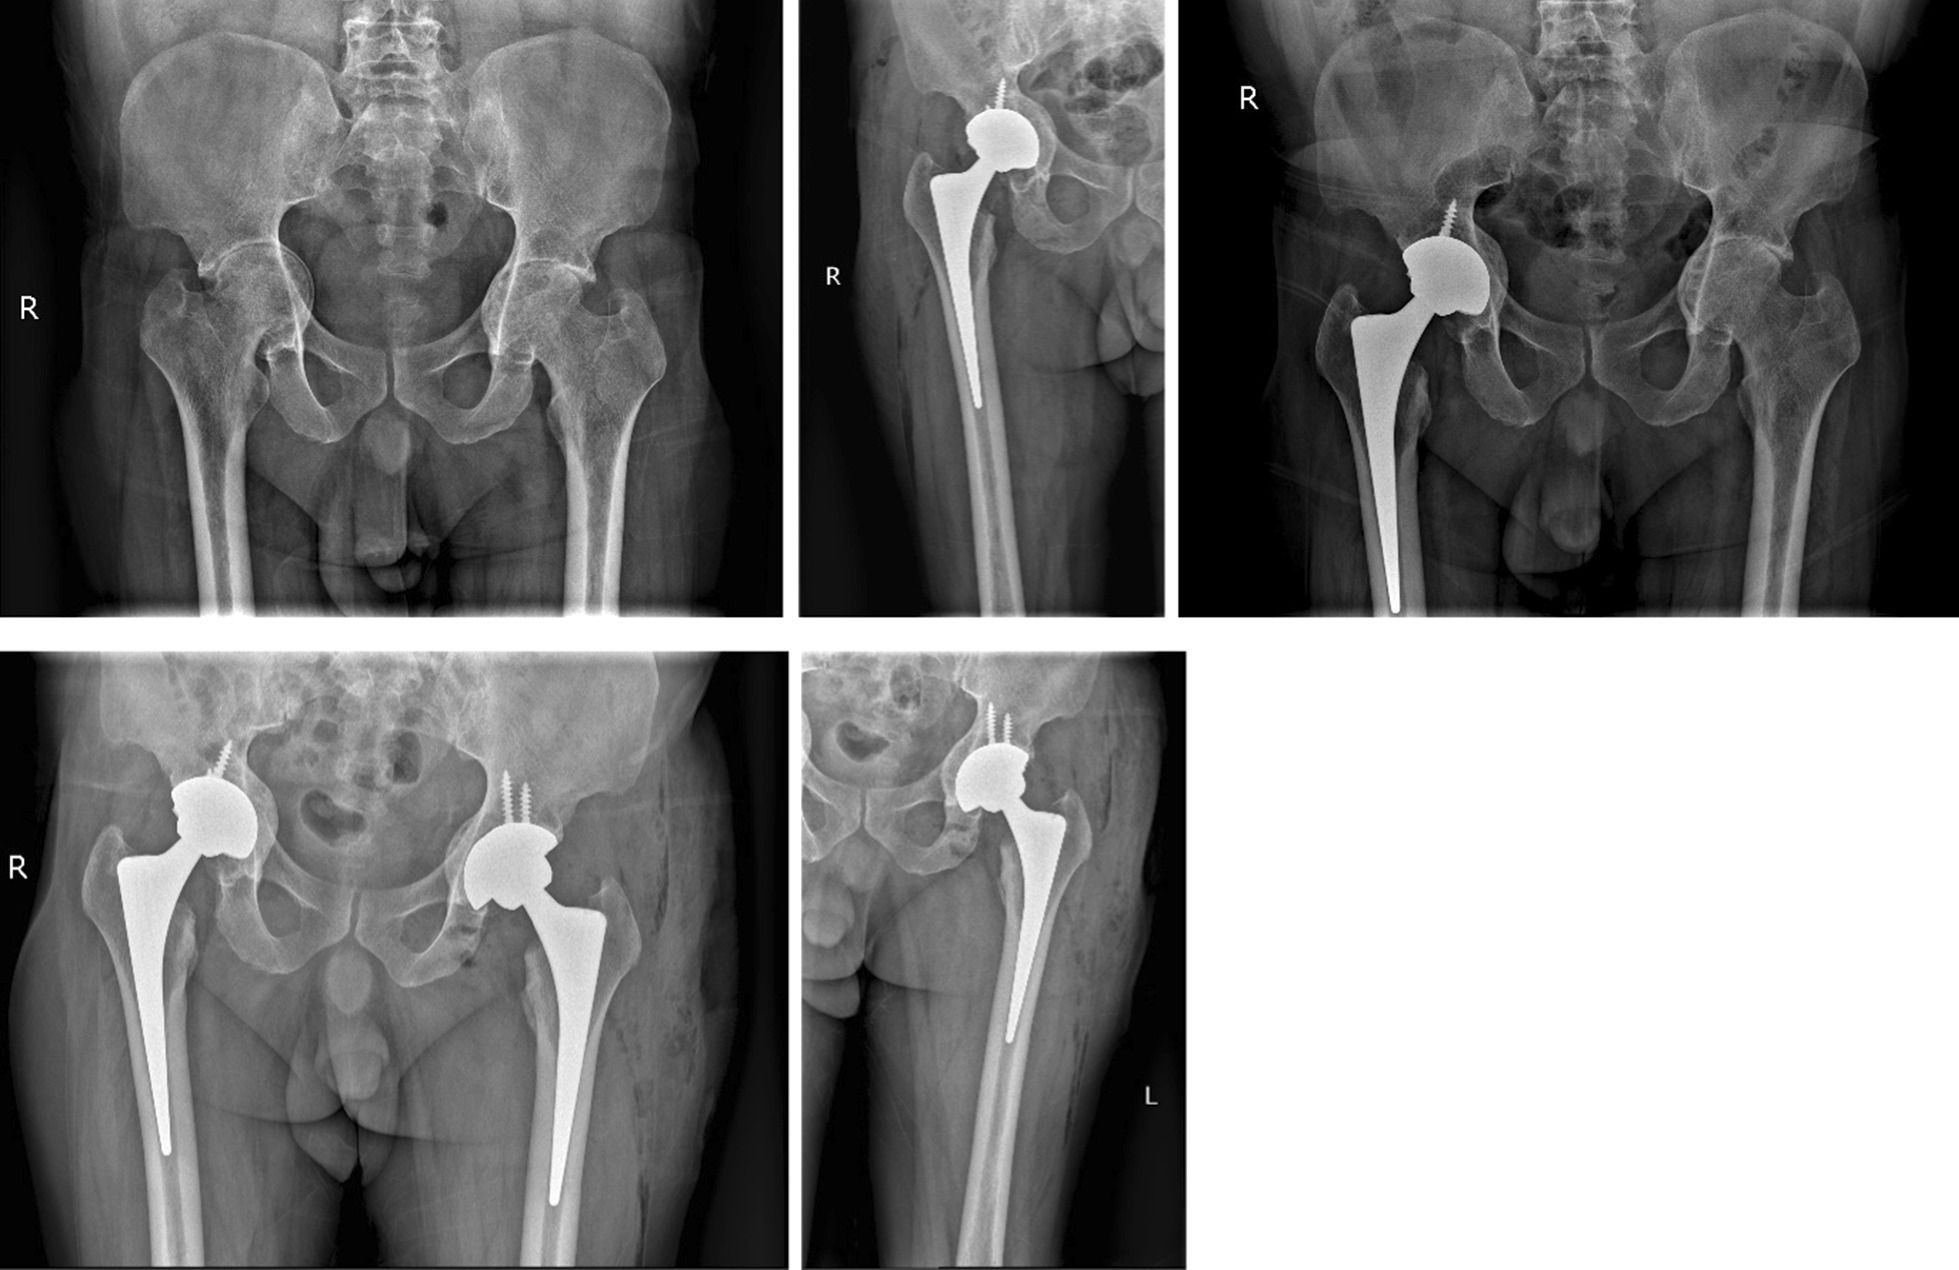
A 54-year-old female patient was diagnosed with bilateral acetabular protrusion secondary to RA. (1) Preoperative anteroposterior x-ray of the pelvis showing bilateral femoral heads protruding inward beyond the Kohler’s line. The patient was classified as type II according to Sotello-Garza and Charnley criterion. (2) Cementless THA accompanied by acetabular reconstruction using impacted bone grafting. Immediate postoperativexX-rays revealed that the acetabular prosthesis had a good position and initial stability, and the rotational center of the hip joint had returned to the normal anatomical location. (3) The 5-year follow-up after right hip replacement, there was no obvious loosening of the prosthesis, invagination of the acetabular cup, complete bone graft healing, and no bone resorption. Anteroposterior x-ray of the pelvis showed that left femoral head protruding inward beyond the Kohler’s line, which was classified as type II. (4) postoperative x-rays revealed that bilateral acetabular prosthesis had a good position and stability, and the left hip center had returned to the normal anatomical location; (5) The 2-year follow-up after left hip replacement, there was no obvious loosening of the prosthesis, and invagination of the acetabular cup
